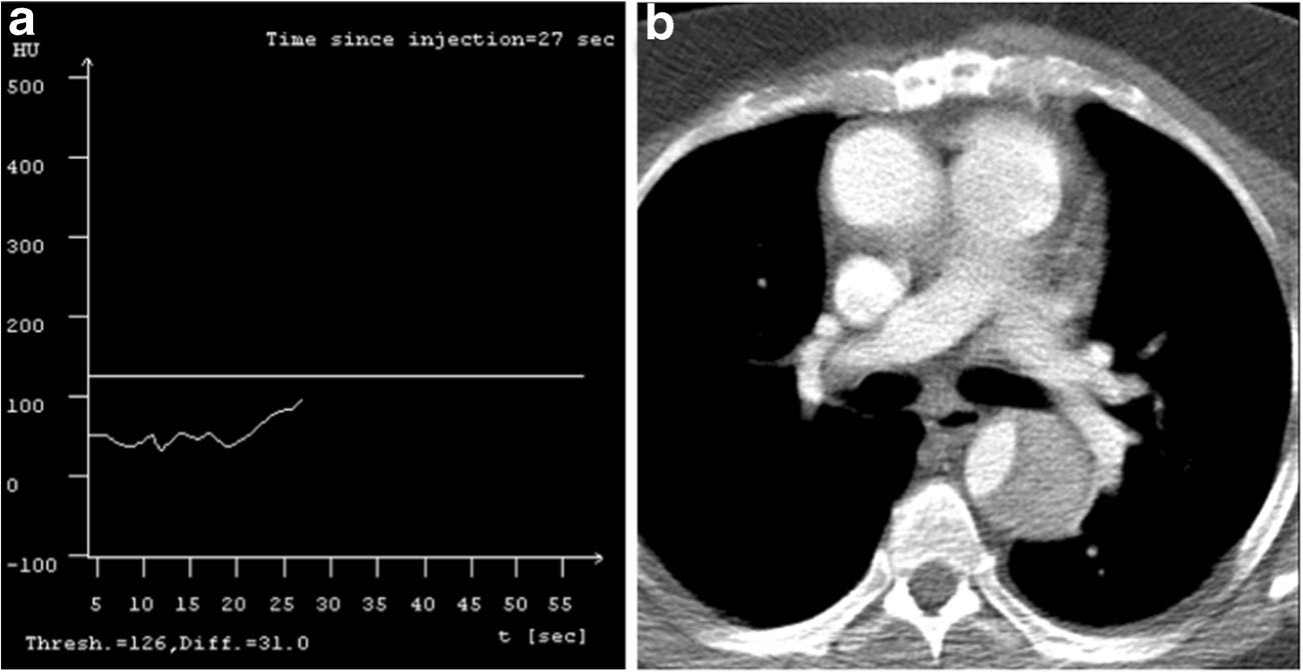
Bolus tracking technique for identification of contrast arrival. Patient with descending aortic dissection with suboptimal increase in contrast opacification within the region of interest (ROI) and failure to trigger the scan (a). The initial CTA ROI was placed in the false lumen (b). The contrast opacification of the false lumen does not reach the threshold required to trigger the scan as seen on on bolus tracker HU-time graph. Repeat injection with placement of ROI in true lumen resulted in adequate evaluation of dissection
